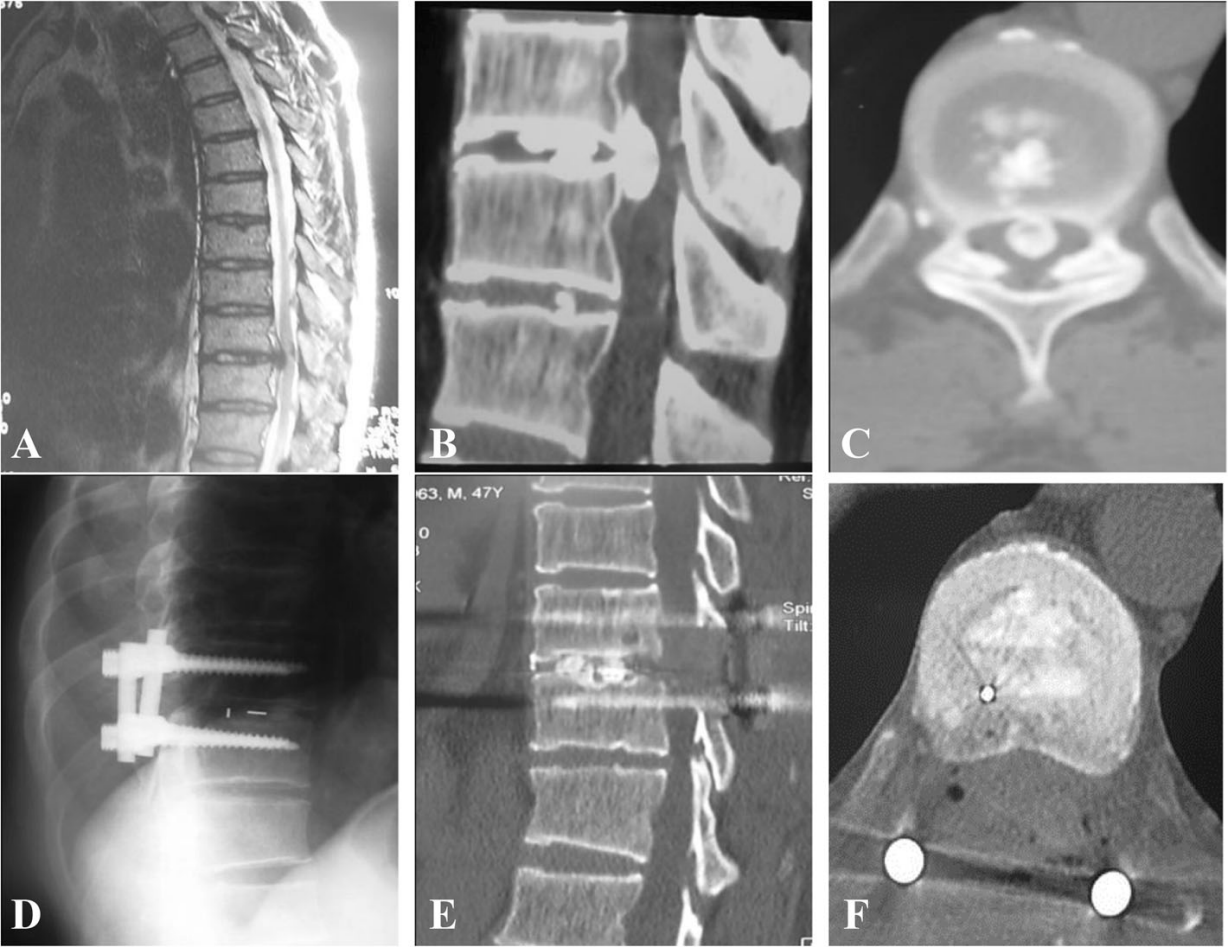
Representative medical images for eggshell procedure. a Preoperative MRI scan showed that there was disc herniation in T9/10 level, and the dura mater was oppressed. b Preoperative sagittal CT scan showed that there was T9/10 disc herniation with calcification. c Preoperative axial CT scan showed that there was T9/10 disc herniation associated with calcification. d Postoperative X-ray showed that the internal fixation was good, and there was intervertebral bone grafting. e, f The postoperative sagittal and axial CT scan showed that the sclerosing herniated disc was removed completely with decompression at 360°
